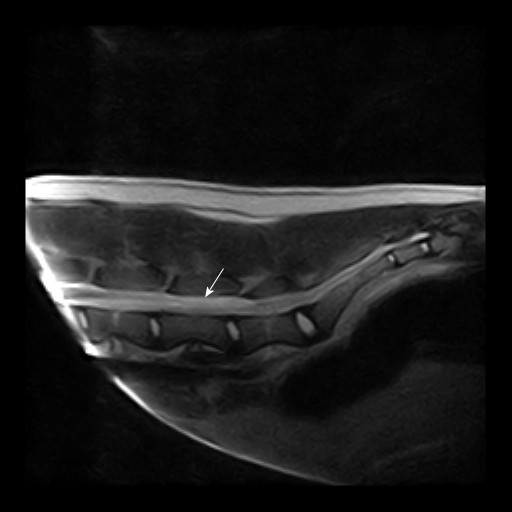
Magnetic resonance imaging of the lumbosacral area, case 4. Sagital T2 weighted images of the lumbosacral spinal cord demonstrating a hyperintense area (arrow) compatible with oedema caudal to the suspected haemorrhage.
